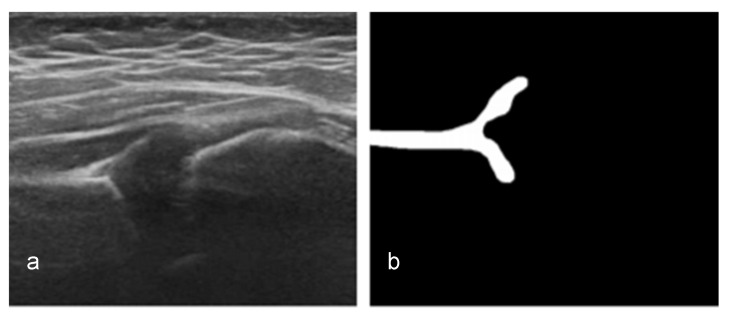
(a) Original image ultrasonographic image of the hip. (b) The same image after the initial conversion.
